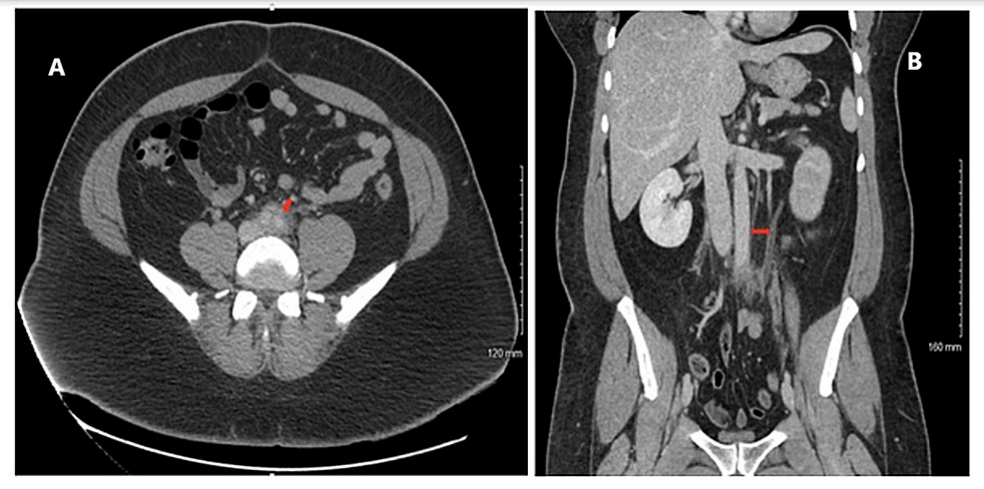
CT abdomen and pelvis with contrast. The red arrow in A shows retroperitoneal fibrosis surrounding the descending vessels originating from the abdominal aorta. The red arrow in B shows left ureteral hydronephrosis before stent placement. CT: computed tomography
